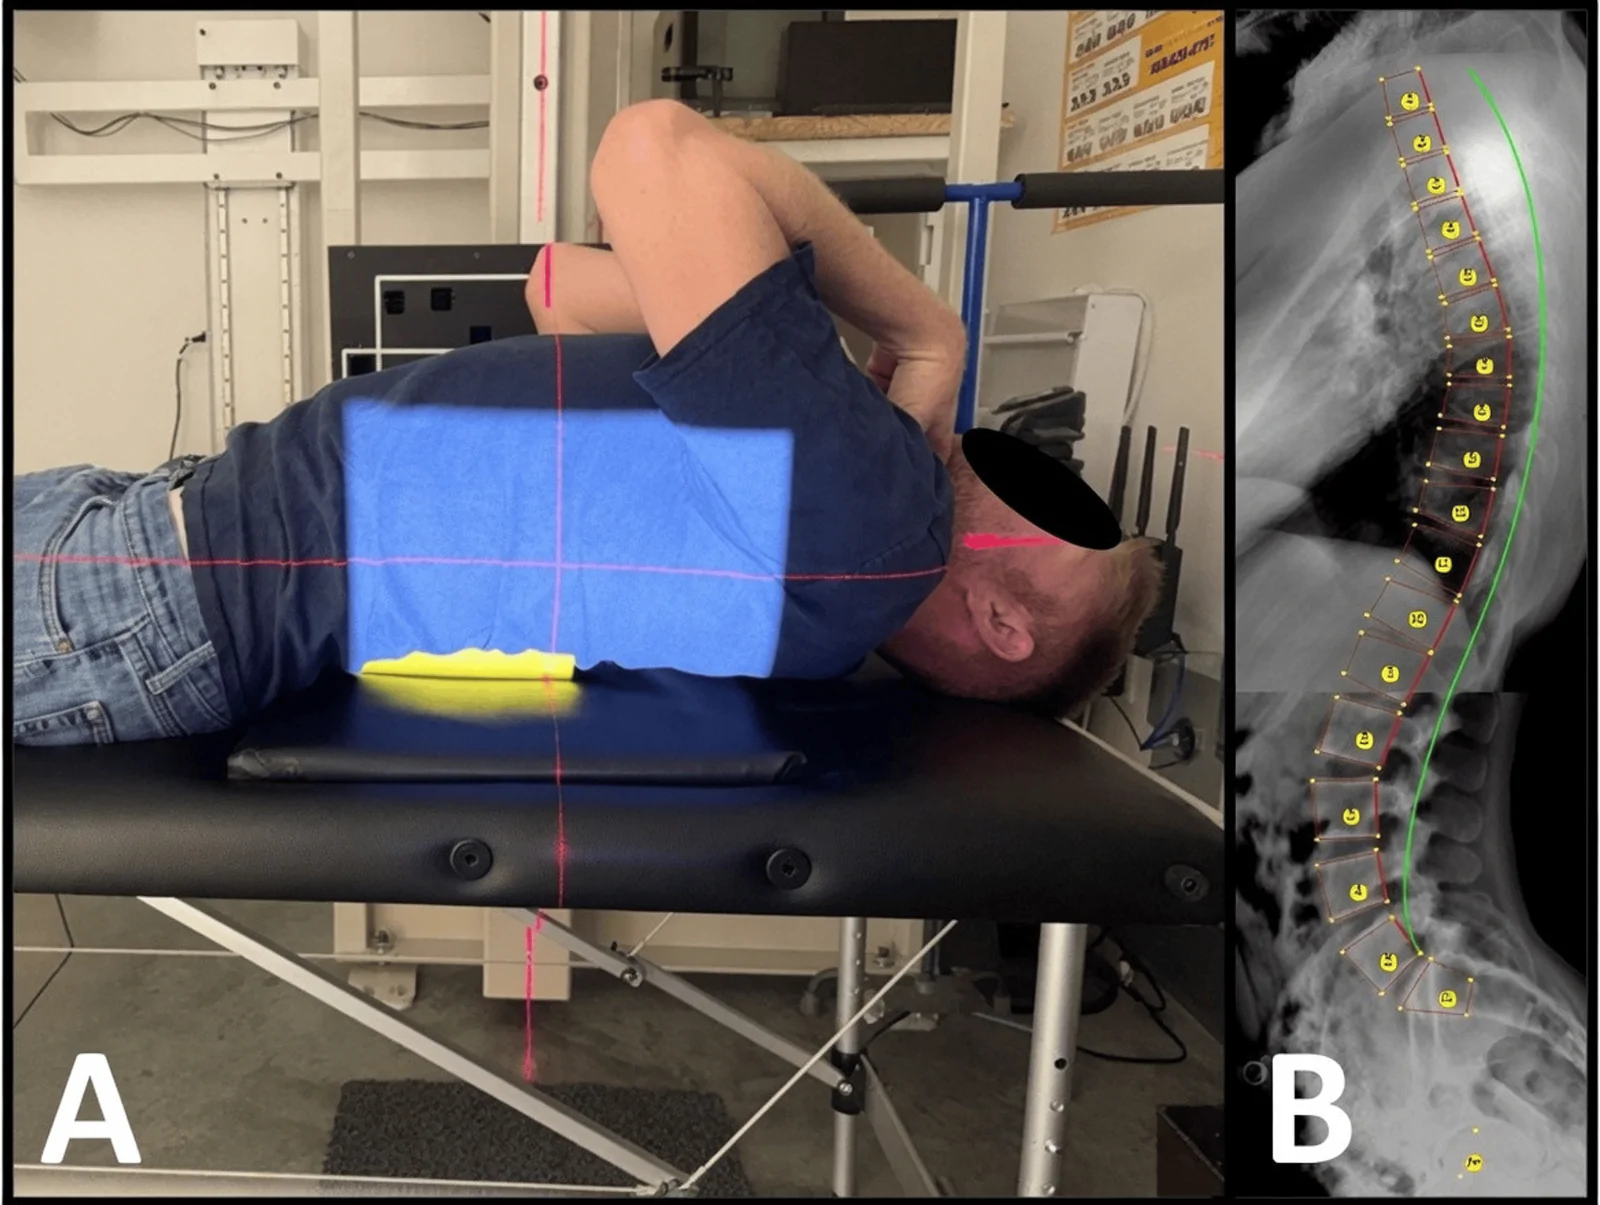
Thoracolumbar spine flexibility supine radiograph with Denneroll(r) and results. (A) Supine radiograph position for thoracic spine flexibility and (B) image results (A) Supine correction potential radiograph with the patient placed in the prone position, with a small, firm foam Denneroll® wedge (TDSO) placed at the apex of the abnormal thoracic kyphosis to induce anterior translation and extension of the thoracic spine. (B) Lateral thoraco-lumbopelvic upright radiograph where the PostureRay® digitization software results are presented as red squares at each margin of the vertebral bodies, as measured by the yellow dots at the anterior-superior, posterior-superior, anterior-inferior, and posterior-inferior points.
